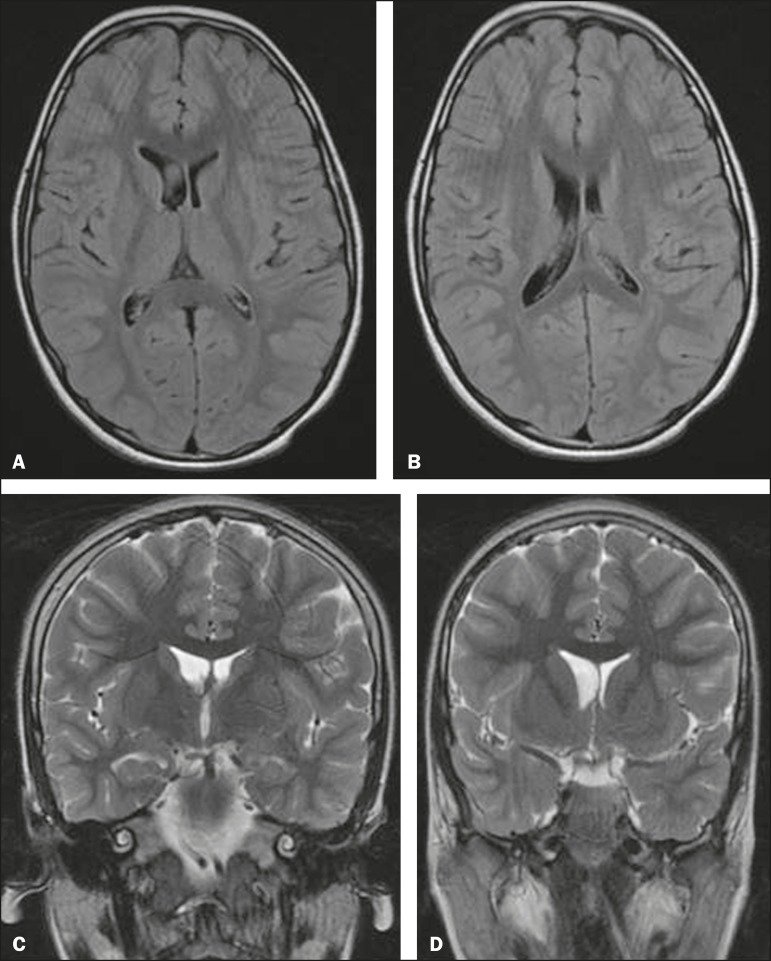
MRI of the brain showing ventricular asymmetry (right lateral ventricle
